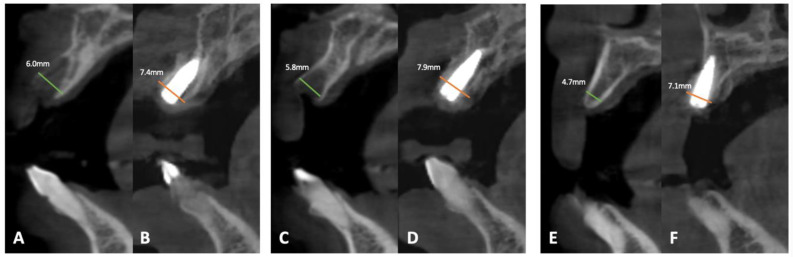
Four-month post-op CBCT evaluation of (A,B) tooth 21, (C,D) tooth 12, and (E,F) tooth 14.
